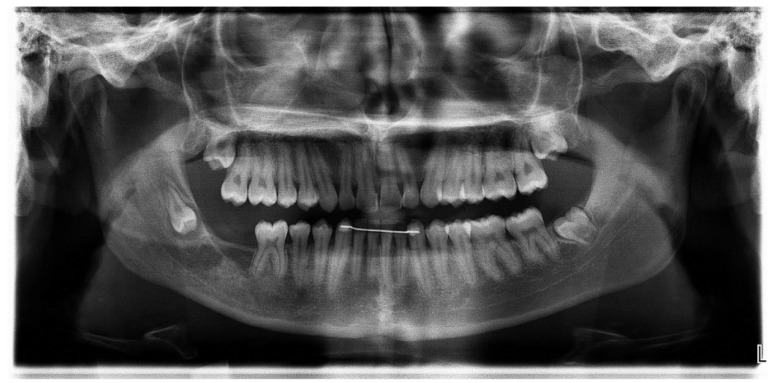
Panoramic radiography one year later: A complete mandible bone healing.
